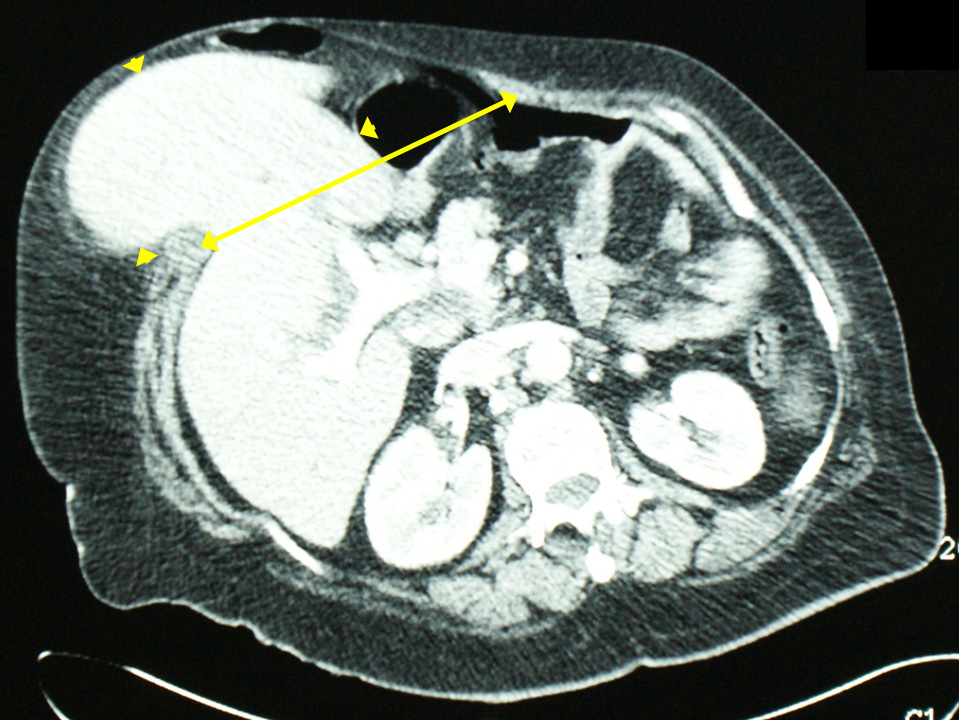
Protrusion of the left hepatic lobe (arrow heads) through the abdominal wall defect (arrow) on axial view of computed tomography.
